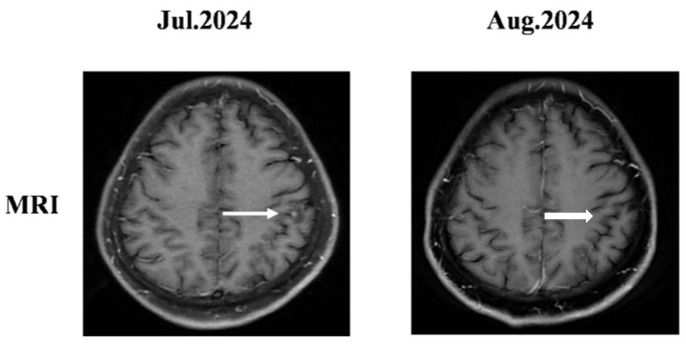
After one month of lorlatinib treatment, the cranial MRI indicated that the metastatic lesion in the left frontal lobe had disappeared. The white arrows indicate the brain metastases.
